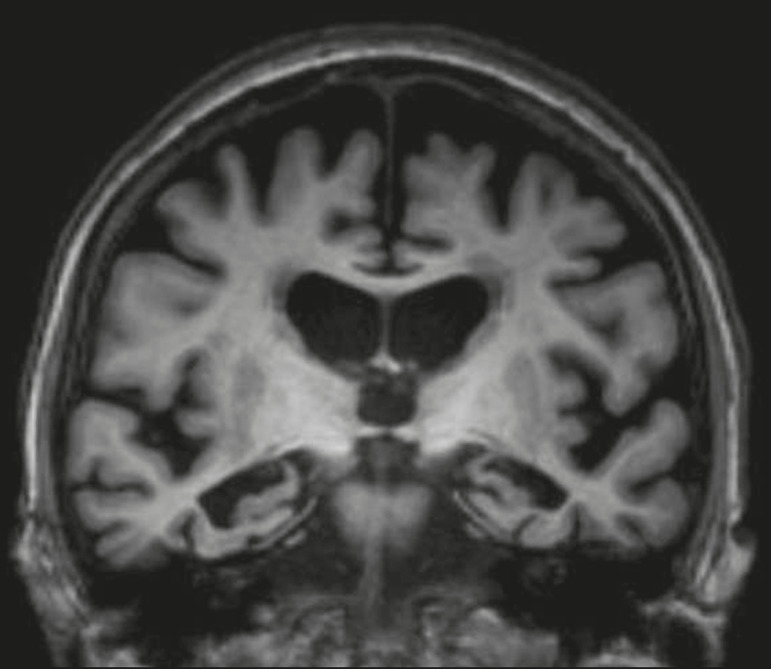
A 77-year-old female patient who presented with short-term memory loss and attention deficit. Coronal T1-weighted MRI sequence showing enlargement of the temporal horns and a reduction in the volume of the hippocampi.
